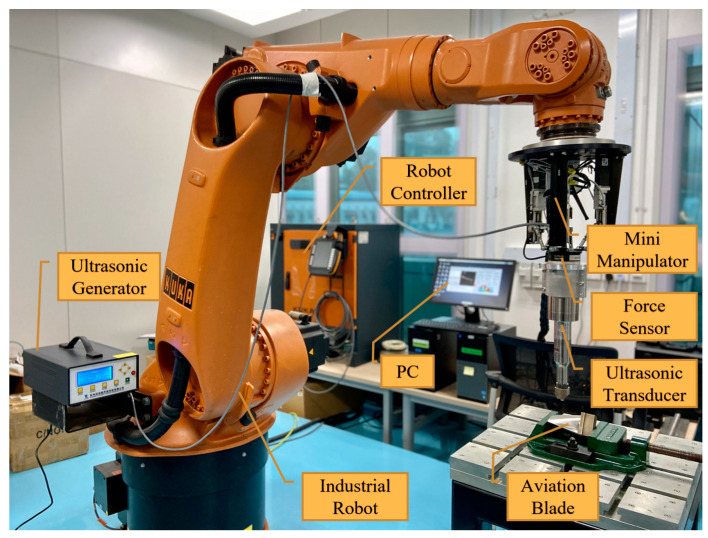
Experimental platform of robotic ultrasonic strengthening.
